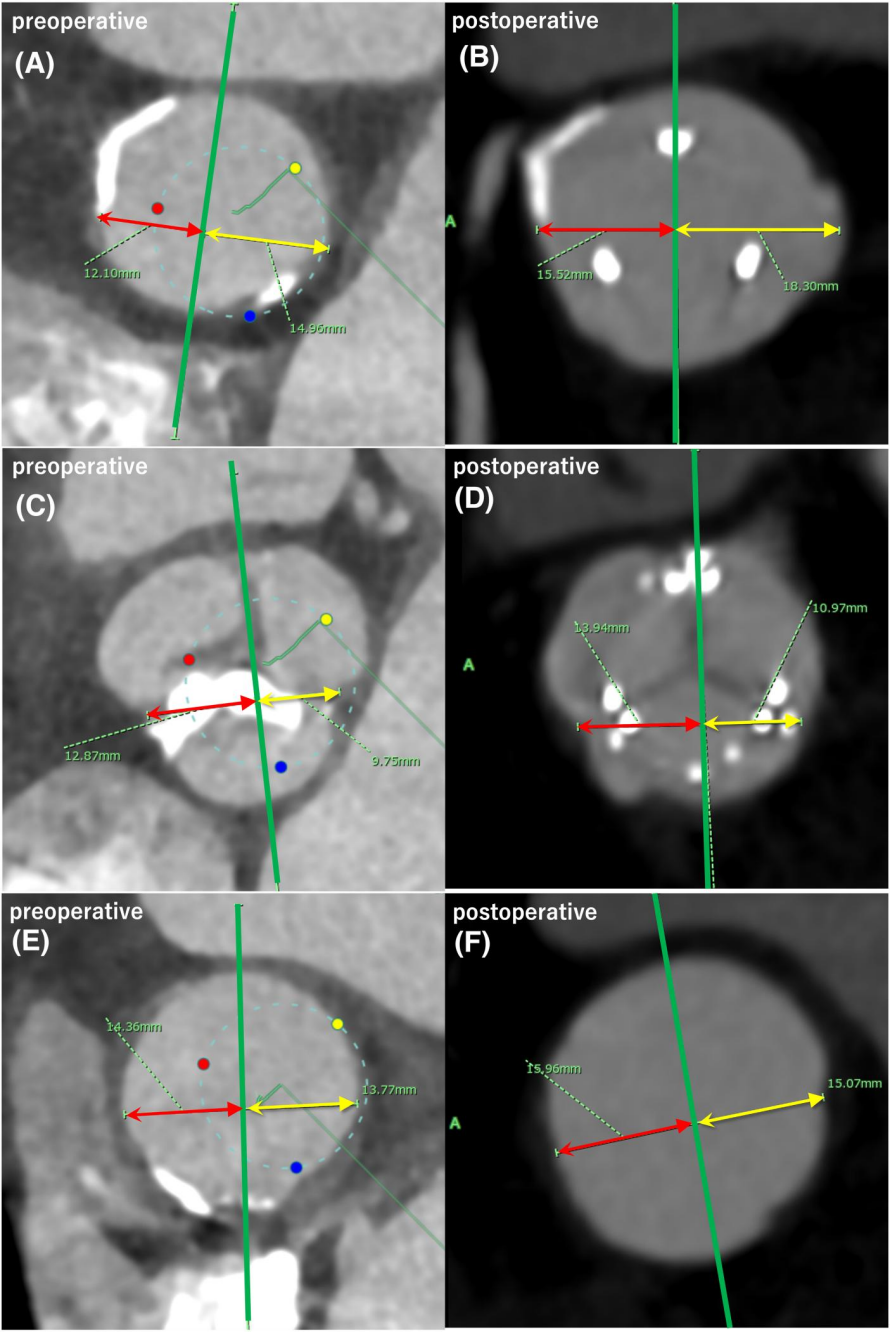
Cardiac Measurements on Computed Tomography Angiography Images. Each Image Pair Indicates Pre- and Postoperative Images, Respectively. (A and B) Sinotubular Junction Level; the Red (Left from Center) and Yellow (Right from Center) Lines Indicate Increases of 3.42 and 3.34 mm, Respectively. (C and D) Valsalva Sinus Level; the Red and Yellow Lines Indicate Increases of 1.07 and 1.22 mm, Respectively. (E and F) Proximal Ascending Aortic Level; the Red and Yellow Lines Indicate Increases of 1.6 and 1.3 mm, Respectively. Green Line: Center Line from the Commissure Between The Left and Right Coronary Sinuses to the Nadir of the Noncoronary Cusp
